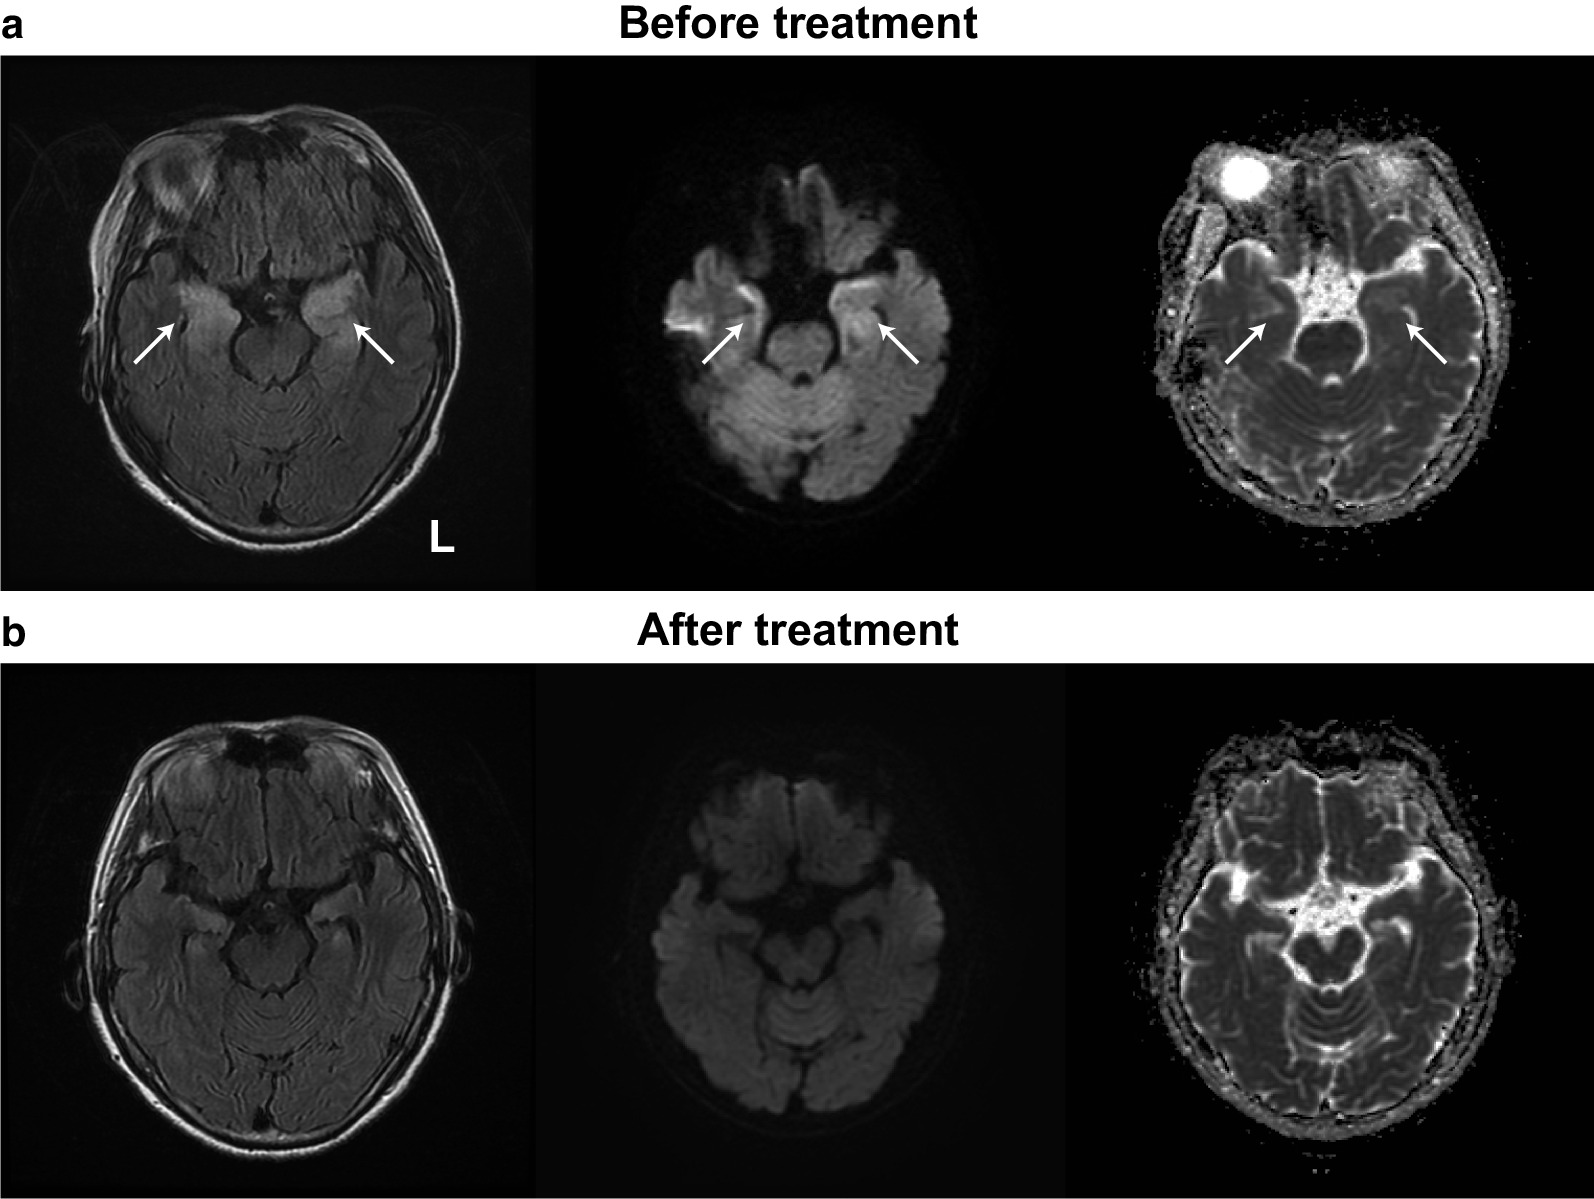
Brain MRI findings. a: The initial MRI (1 week after admission). Before treatment, brain MRI showed abnormal hyperintensity areas in the bilateral medial temporal lobes (arrows) on FLAIR sequence (left). Diffusion-weighted imaging (middle) and apparent diffusion coefficient map (right) also show abnormal hyperintensity in these regions. b: Follow-up MRI (2 months after admission). After treatment, the abnormal hyperintensity in the initial MRI was resolved
